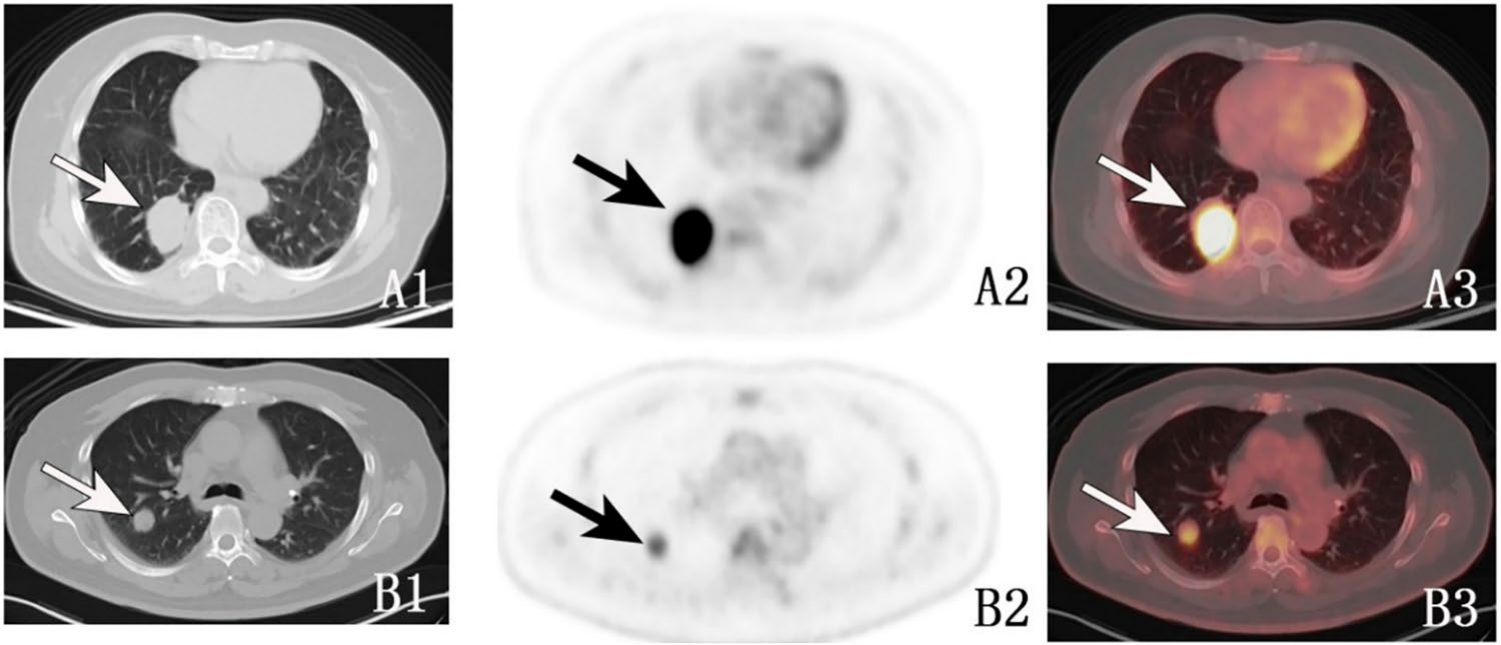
A The axial PET/CT images of a 71-year-old female patient, 96 months post-resection of cervical cancer, reveal a solitary lung mass in the right lower lobe (A1 arrow: axial CT image, dimensions: 30 mm × 39 mm) and significant hypermetabolism of glycose (A2 and A3 arrows: axial PET and PET/CT images, SUVpeak: 15.8, TLG: 193.6). The pathological diagnosis was SPLC. B The axial PET/CT images of a 50-year-old male patient with colon cancer. Simultaneously, a solitary lung nodule was found in the right upper lobe (B1 arrow: the axial CT image, size: 19 mm × 20 mm) as well as hypermetabolism of glycose (B2 and B3 arrows: the axial PET and PET/CT images, SUVpeak: 3.8, TLG: 12.6). The pathological diagnosis was PM
